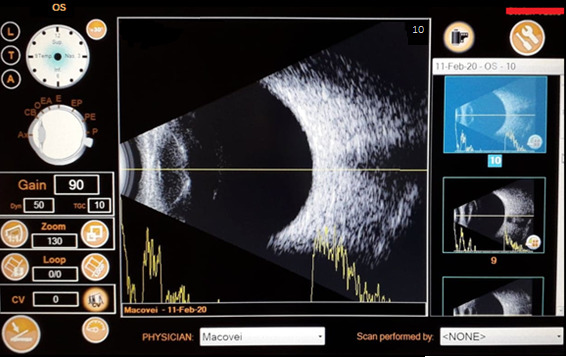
Ultrasound imaging LE: axial scan
